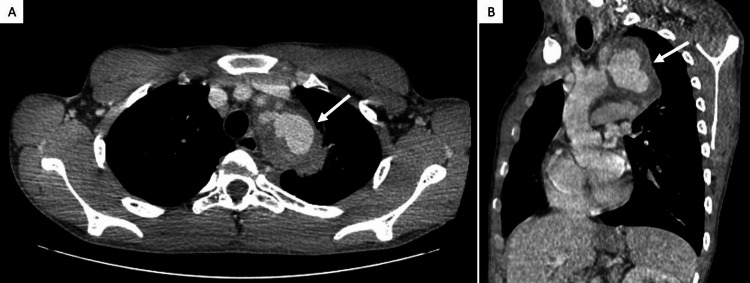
CT angiography revealing left subclavian artery pseudoaneurysm (white arrows) (A) Axial view and (B) sagittal view
